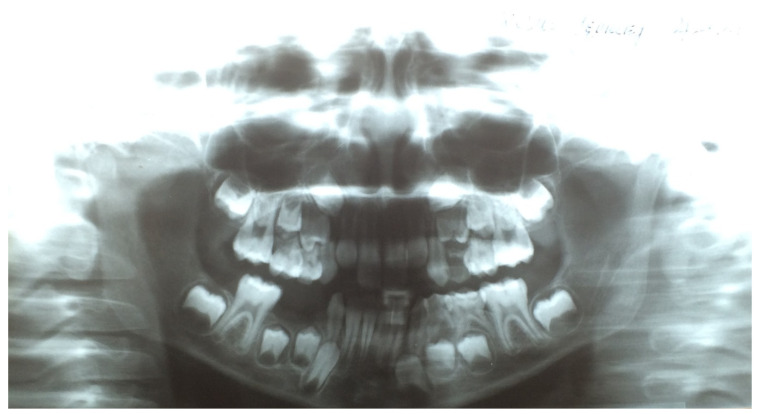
Pantomographic radiograph of an 8-year-old girl.
